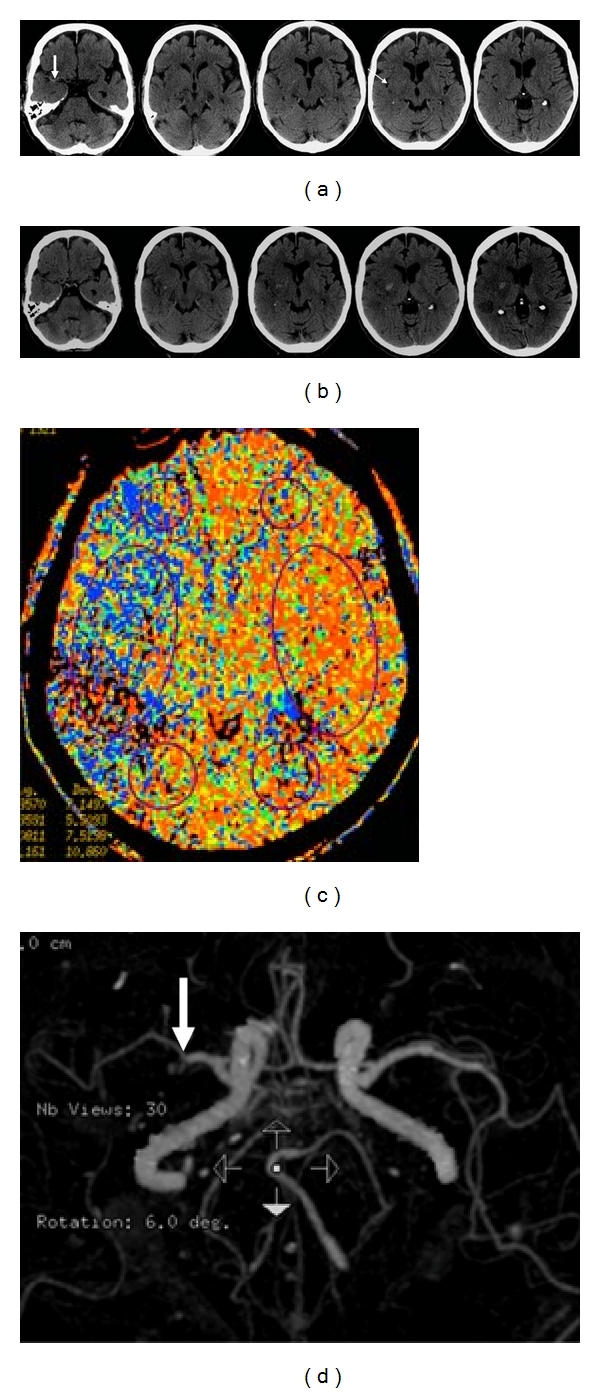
82-year-old man presented with left hemiparesis. (a) Axial unenhanced CT images 2 hours after stroke show a hyperdense right MCA (thick arrow) and loss of the insular ribbon (thin arrow). Patient received intravenous thrombolysis after the CT. (b) Axial unenhanced CT images 3 days later show petechial hemorrhages in the striatocapsular area and a hematoma within the right lentiform nucleus, consistent with hemorrhagic transformation after thrombolysis. Stage HI d1 [1]. (c) CT perfusion image shows a large area of delayed mean transit time on the right. (d) CT angiogram shows truncation of mid M1 segment of the right middle cerebral artery (arrow).
